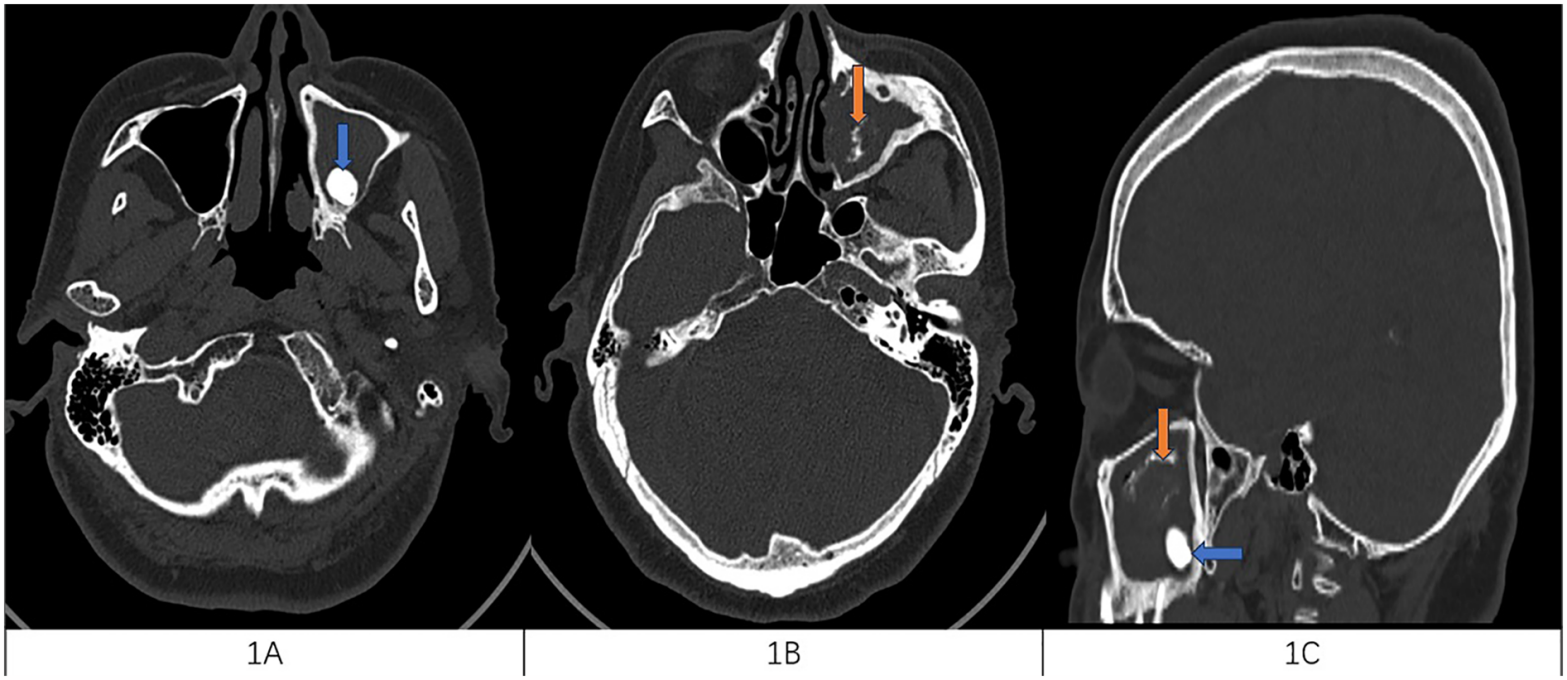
(A) Horizontal CT scan of the sinuses with a blue arrow on the left side of the maxillary sinus with high-density mass considering the ectopic tooth. (B) The orange arrow on the horizontal sinus CT shows a high-density cord image of the left maxillary sinus, considering fungal infection. (C) Both lesions were present on the CT disfigurement of the sinuses orange for fungal infection and blue for ectopic teeth.
